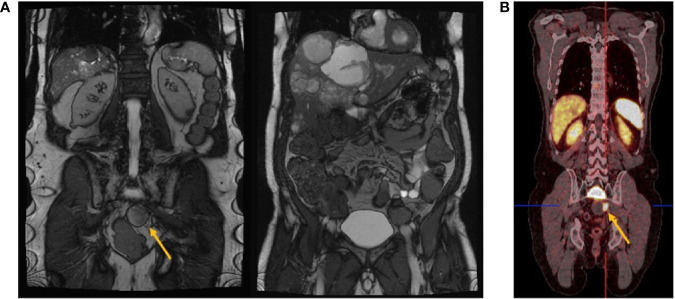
(A) Magnetic resonance imaging. Coronal view demonstrating primary presacral neuroendocrine neoplasm (yellow arrow) and liver metastases. (B) Coronal view of a 68Ga-DOTATOC-PET/CT scan showing SSTR expression of the whole body. While the liver metastases showed a homogeneous SSTR expression, only a part of the presacral lesion showed a homogeneous SSTR expression (yellow arrow), suggesting a SSTR-negative/cystic portion besides the SSTR-positive solid presacral NEN. MRI and 68-DOTATOC-PET/CT are from the same patient (study-ID III) at different time points.
